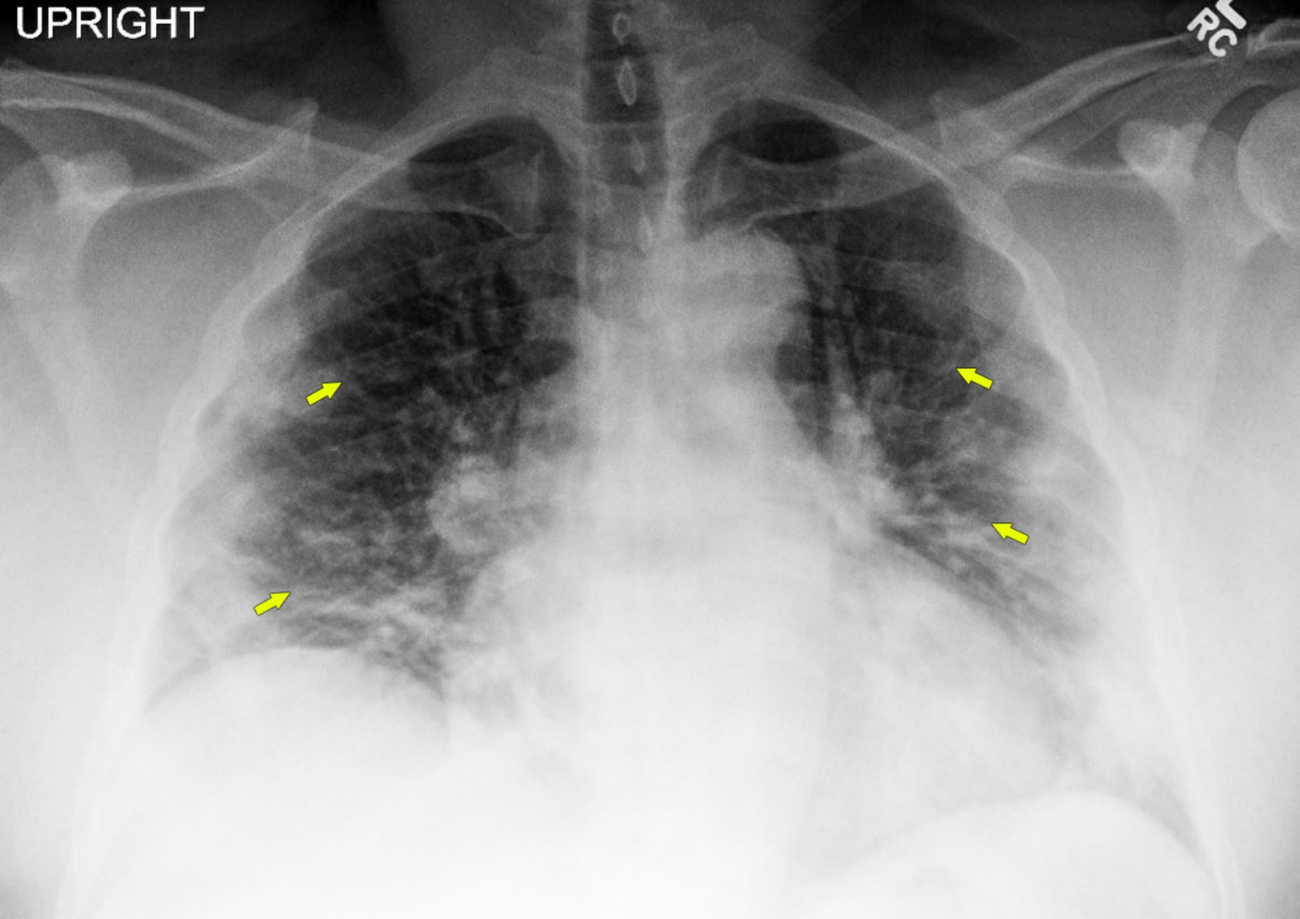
Chest x-ray showing bilateral patchy peripheral infiltrates (arrows).
